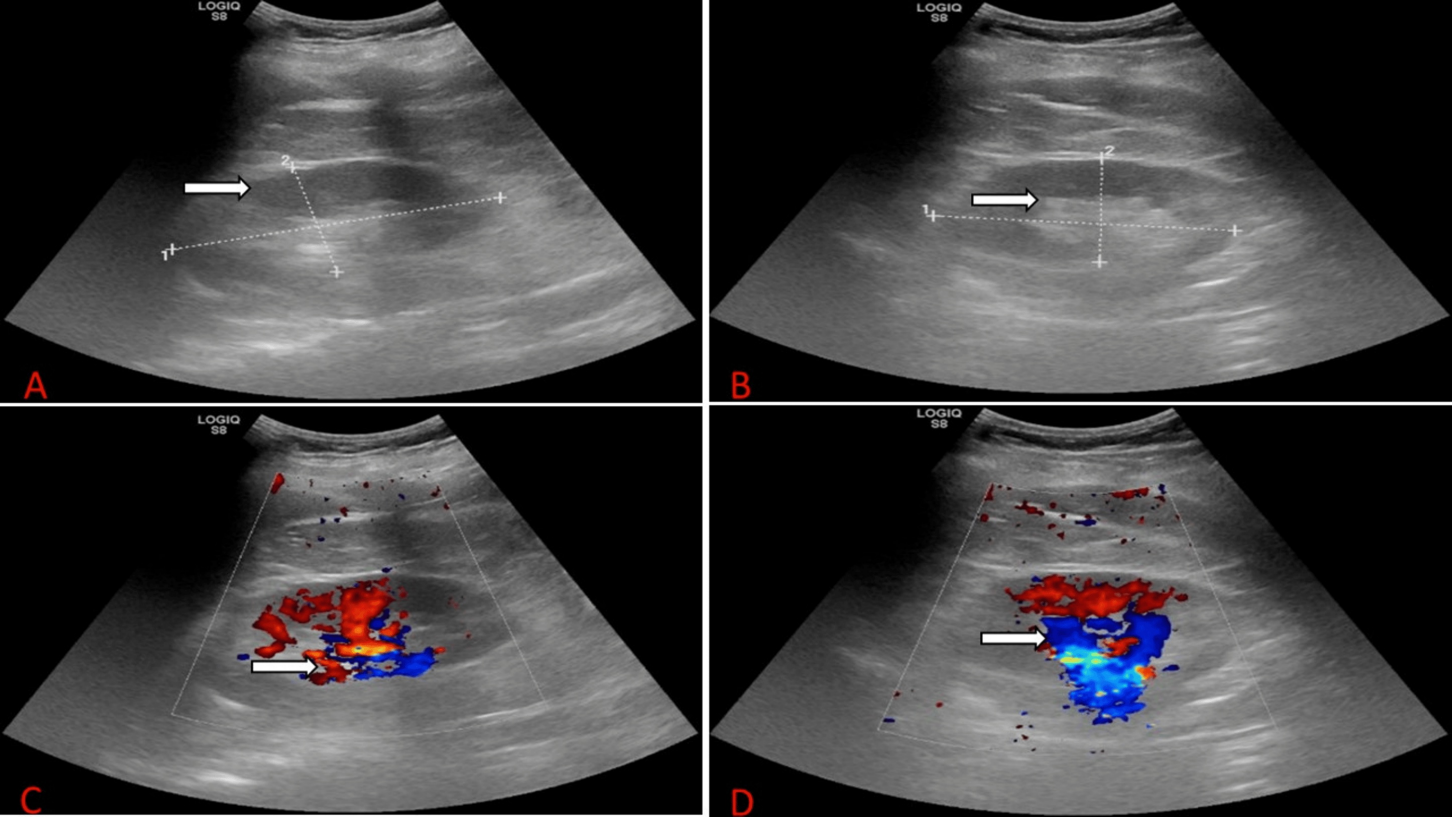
The kidney ultrasound done in 2024. A: The right kidney measuring 11.8 × 5.0 × 5.9 cm with mildly increased corticomedullary echogenicity (arrow) but normal parenchyma thickness and contour, no pelvicalyceal dilatation, no calculi, no cysts, and no solid masses. B: The left kidney measuring 10.0 × 4.8 × 5.0 cm with mildly increased corticomedullary echogenicity (arrow) but normal parenchyma thickness and contour, no pelvicalyceal dilatation, no calculi, no cysts, and no solid masses. C: Kidney ultrasound with Doppler images (arrow) of the right kidney. D: Kidney ultrasound with Doppler images (arrow) of the left kidney.
